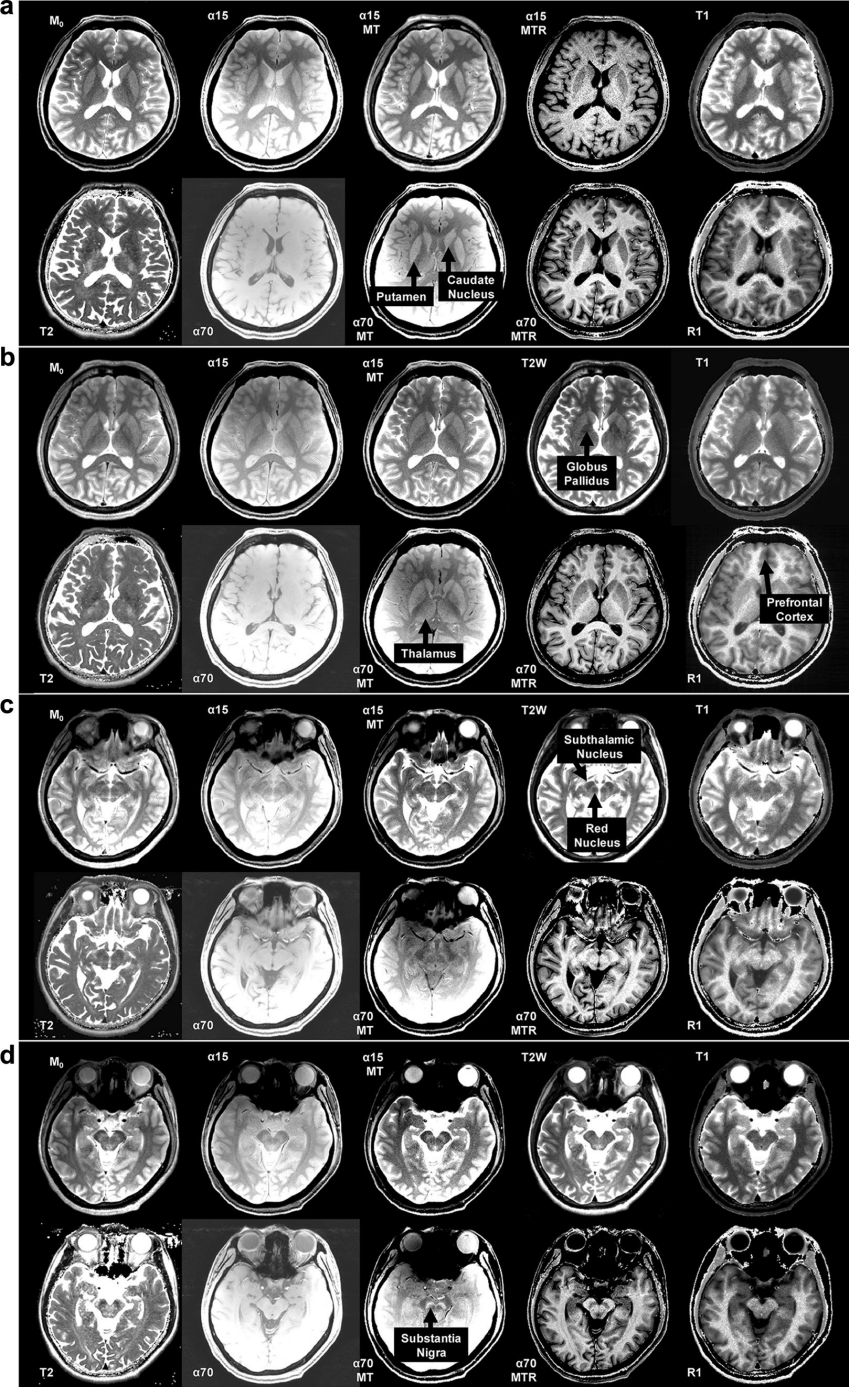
Magnetization transfer MRI provides proton-density contrast in brain while saturating long-*T*_1_ extracellular protons. a Transversal *M*_0_ map (*M*_0_), proton-density-weighted MRI without (*α*15) and with MT (*α*15 MT), MT ratio map (*α*15 MTR), *T*_1_ map (*T*_1_), *T*_2_ map (*T*_2_), *T*_1_-weighted MRI without (*α*70) and with MT (*α*70 MT), MT ratio map (*α*70 MTR), and *R*_1_ map (*R*_1_) showing the caudate nucleus and putamen, b globus pallidus, thalamus, and prefrontal cortex, c subthalamic and red nuclei, and d substantia nigra. Note the similar contrast for *M*_0,_*α*15 MT, and *α*70 MT except for cerebrospinal fluid
